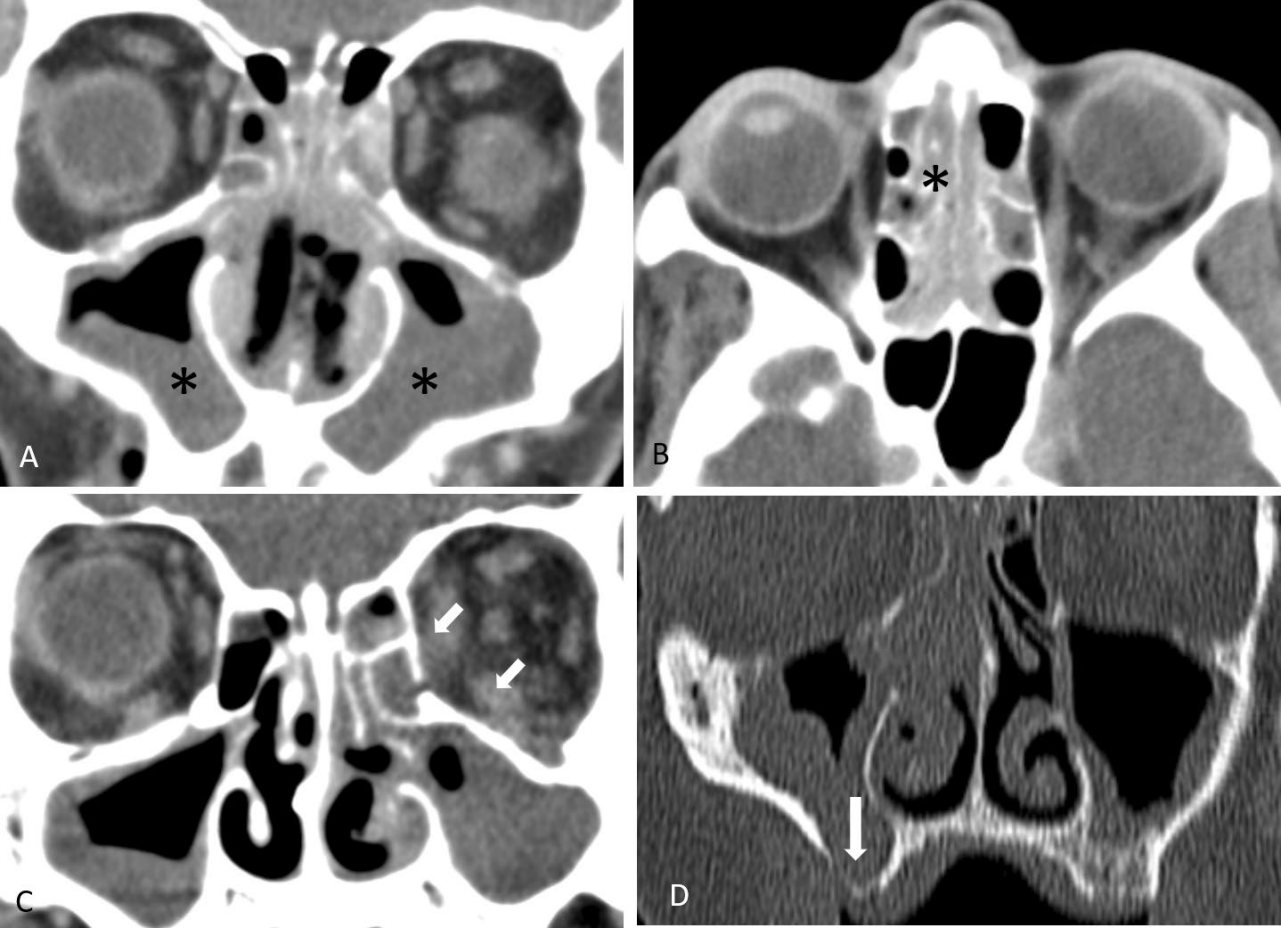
Examples of findings in patients with *Mucor* AIFR. (A) Coronal CT image shows bilateral mucosal thickening involving the maxillary sinuses (asterisks) and the nasal cavity. (B) Axial image in the same patient demonstrates bilateral mucosal thickening of ethmoid air cells (asterisk). (C) Coronal image in a different patient shows bilateral maxillary sinuses involvement and left orbital involvement with fatty infiltration of the left medial and inferior extraconal orbital fat (arrows). (D) Coronal CT of a different patient shows bilateral mucosal thickening of the maxillary sinuses with bony dehiscence along the inferior aspect of the right maxillary sinus.
